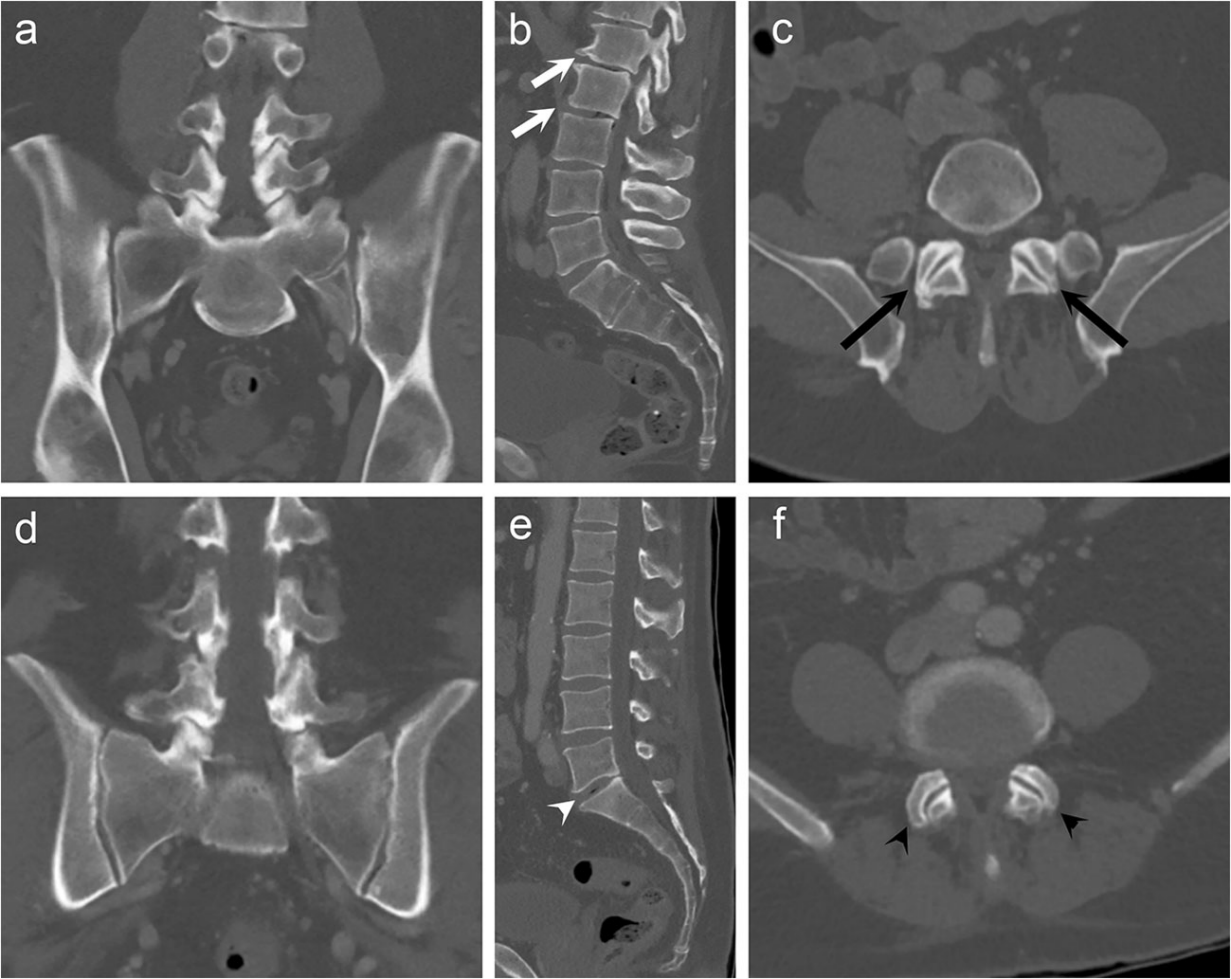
General lumbar degeneration is associated with lumbosacral transitional vertebra (LSTV). Coronal CT image (a) of a 64-year-old male with a type IV LSTV describing moderate degeneration at upper lumbar spine (white arrows) in sagittal view (b) and at L4/5 facet joints (black arrows) in axial view (c). Coronal CT image (d) of a 64-year-old male without LSTV showing only mild disc degeneration at L5/S1 (white arrowhead) in sagittal view (e) and at L4/5 facets (black arrowheads) in axial view (f)
